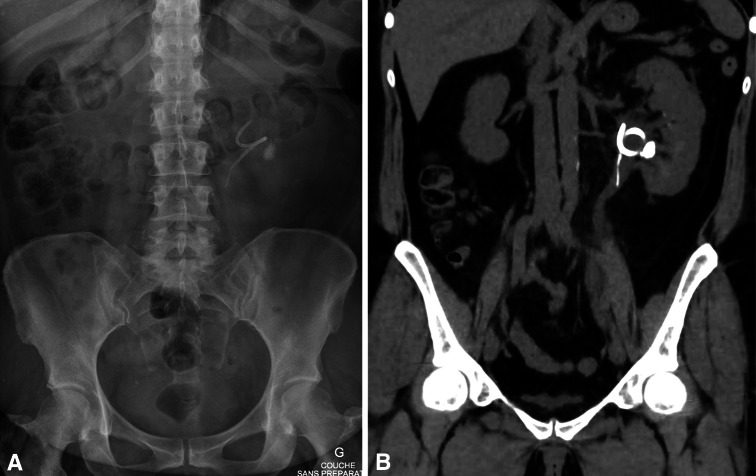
Patient with left renal stone and PSS. a Appearance of the PSS and the stone on X ray. b Dilated ureter on CT scan 1 month after PSS implantation
